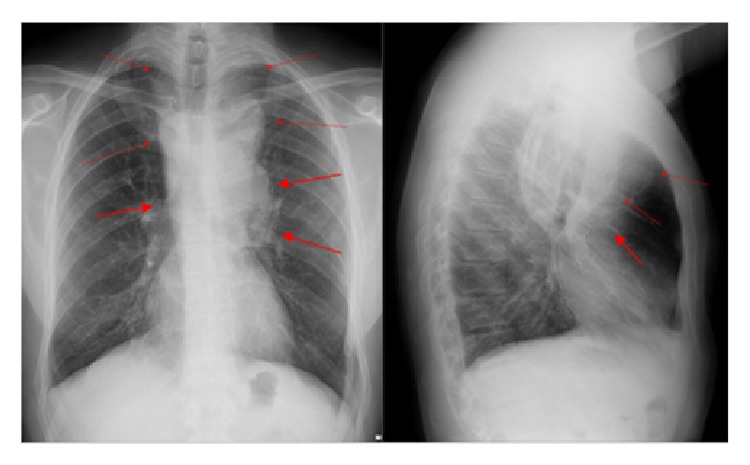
Chest X-ray in PA and Profile shows enlargement of the mediastinum (red arrows).
